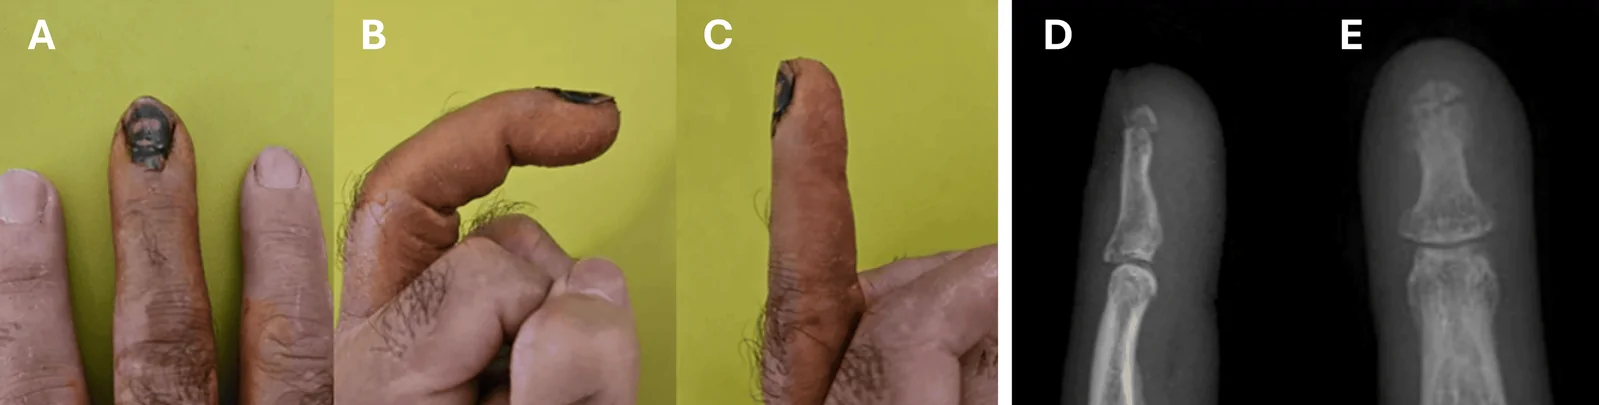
Comprehensive six-week follow-up and multi-planar assessment. Clinical photographs (left panel) and corresponding radiographs (right panel) at six weeks post-injury and two weeks following needle removal. Healed soft tissues and nail plate are evident in all clinical views. Active joint motion is demonstrated. Radiographic alignment is anatomical in both planes. (A) Dorsal view of fisted hand. (B) Proximal lateral view of fisted hand. (C) Close-up lateral profile view of the third digit fingertip. (D) Standard lateral radiograph. (E) Standard anteroposterior (AP) radiograph.
